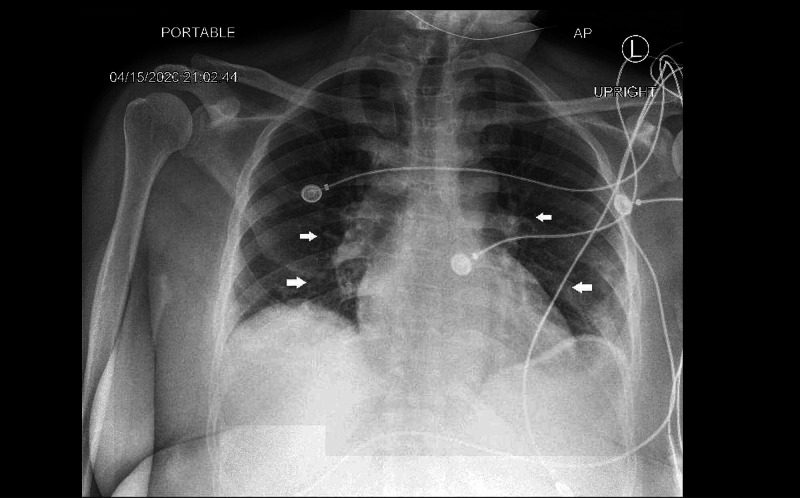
Chest x-ray showing patchy bilateral infiltrates (arrows)
